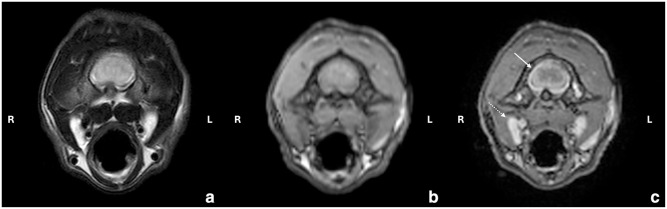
Transverse (a) T2-weighted, (b) T1W precontrast and (c) T1-weighted (T1W) postcontrast images of the cervical spinal cord at the level of C1. The meninges are moderately thickened with strong contrast enhancement (white arrow), which is compatible with marked meningitis. Mild bilateral medial retropharyngeal lymphadenomegaly (dashed white arrow)
